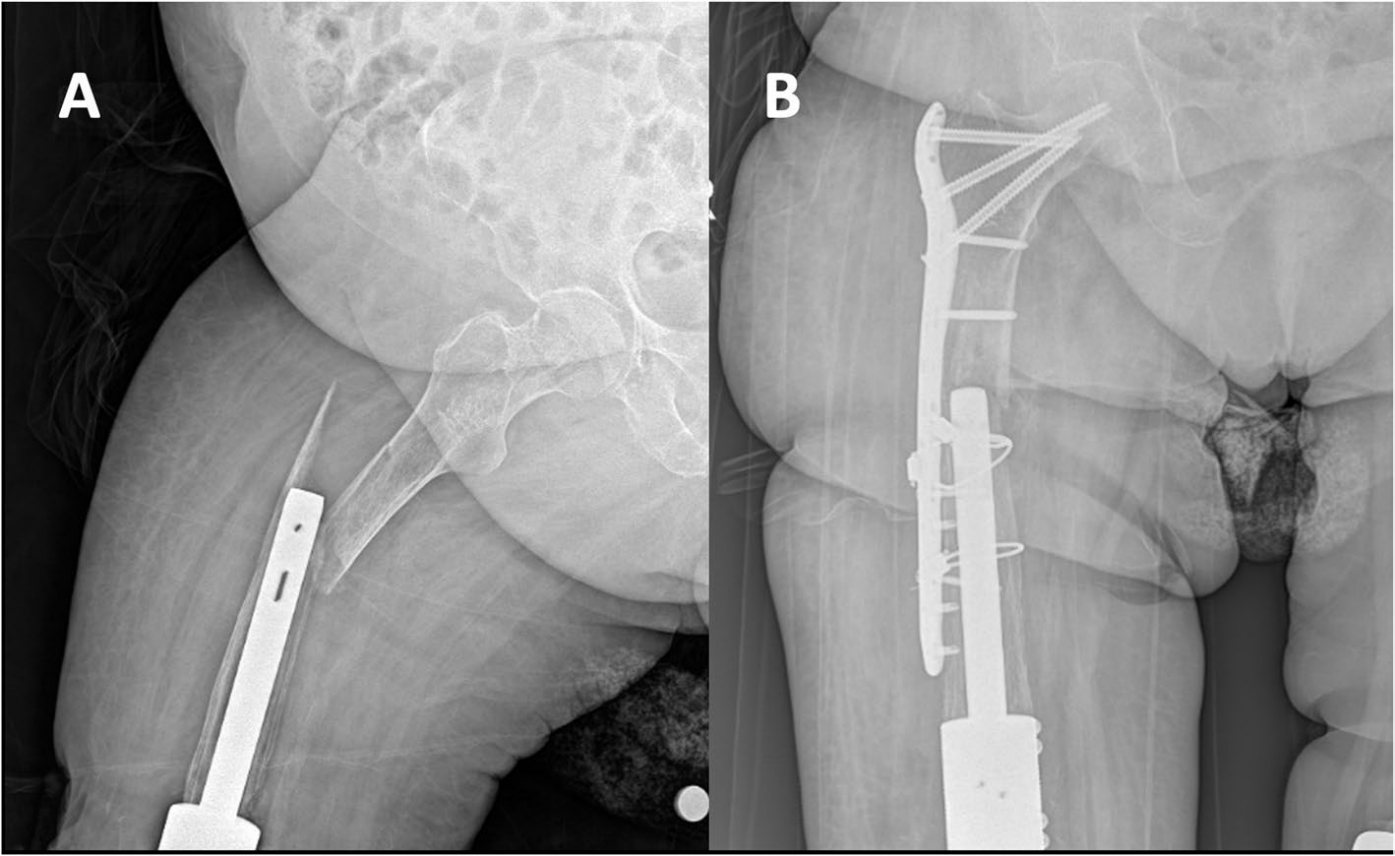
a Radiographs depicting a periprosthetic fracture in proximal femur after knee arthrodesis and (b) internal fixation of fracture with a plate
